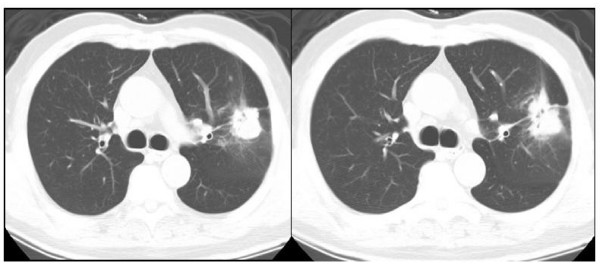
Computed tomography chest scan at initial visit. Lung and mediastinal windows show cavitary mass involving both left upper and lower lobes across the major fissure. A calcified ipsilateral lymph node can be seen in the mediastinal windows. This lesion and various lymph nodes were subsequently shown to be positive by positron emission tomography.
